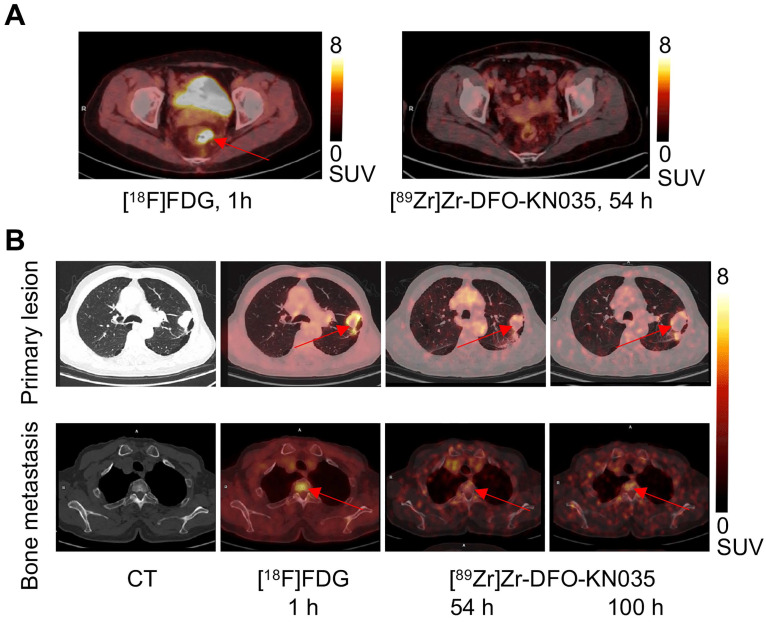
Comparison of the [^18^F]FDG and [^89^Zr]Zr-DFO-KN035 PET imaging of cases negative (No. 11) and positive (No. 2) for PD-L1. (A) Representative PET/CT imaging of transverse section of colorectal cancer in the same PD-L1-negative patient acquired by using [^18^F]FDG (1 h) and the PD-L1-targeting molecular-imaging tracer [^89^Zr]Zr-DFO-KN035 (54 h). (B) Representative CT and PET/CT images of layers of lung primary cancer (top lane) and one bone metastasis site (bottom lane; thoracic vertebrae 2) acquired by using [^18^F]FDG (1 h) and [^89^Zr]Zr-DFO-KN035 (54 and 100 h) in a PD-L1-positive patient. Red arrows indicate the locations of primary cancer and bone metastatic site.
